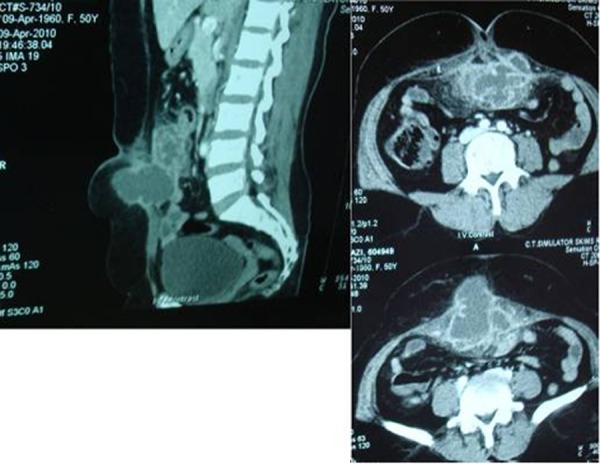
CT scan showing abdominal wall abscess and adjacent bowel walls.
